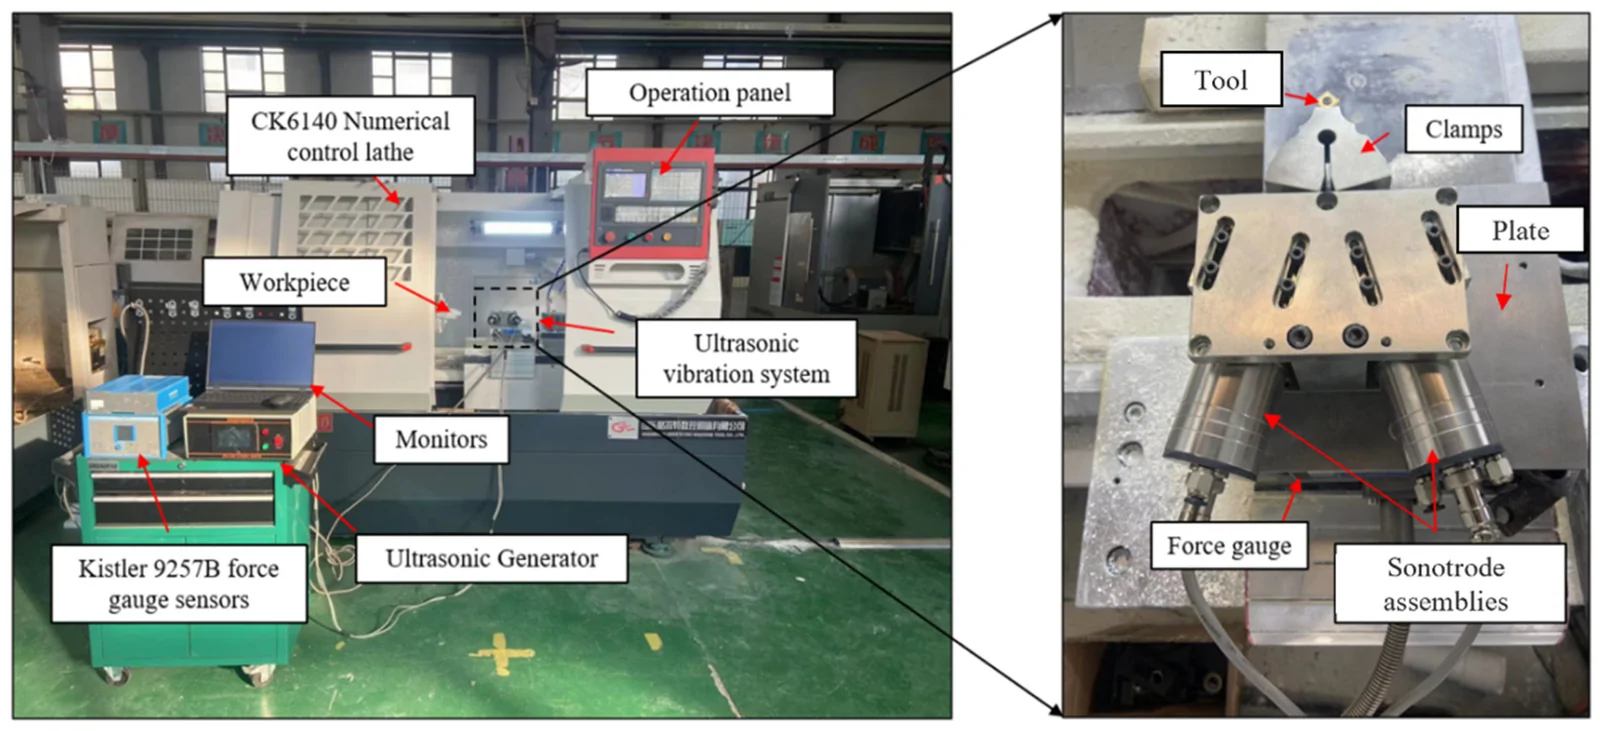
Ultrasonic vibration cutting test platform.
